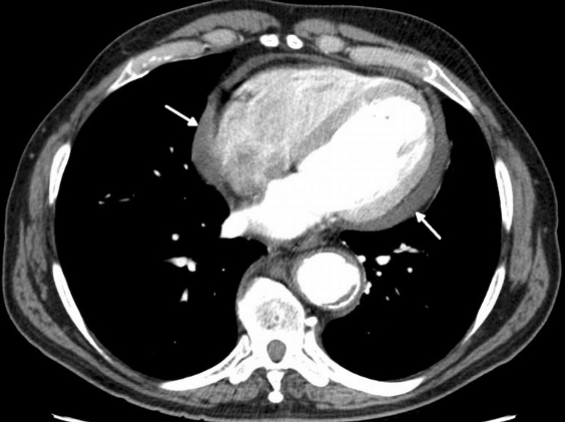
Axial enhanced CT images of the thorax in a 73-year-old male showing hypodense soft tissue infiltration of the pericardium (arrows)
